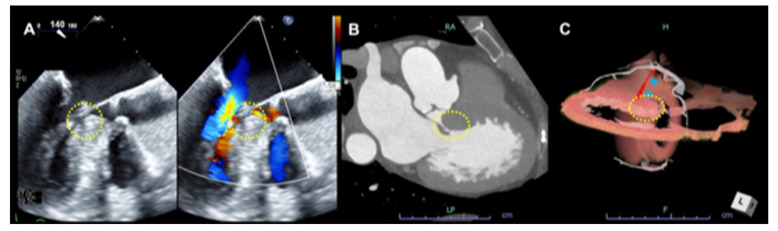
A representative case of LVOT obstruction. Transesophageal echocardiography (A), CT images for LVOT obstruction (B), and a target septal branch for PTSMA (C).
